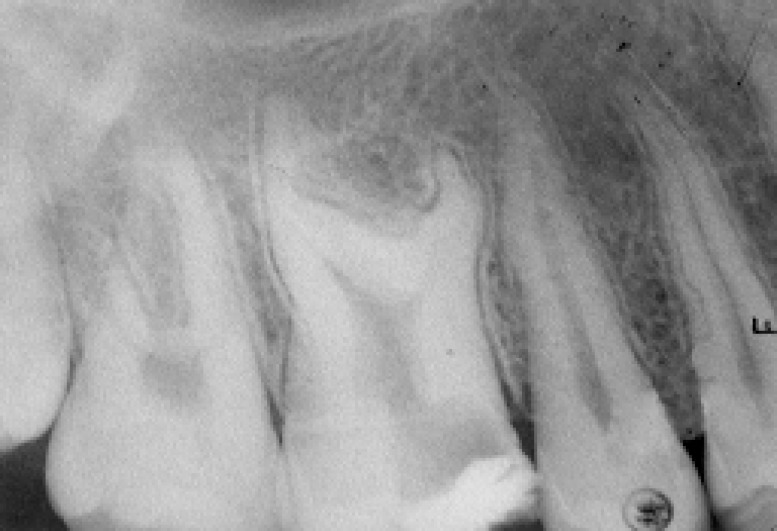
Periapical radiographic images of right maxillary first molar
